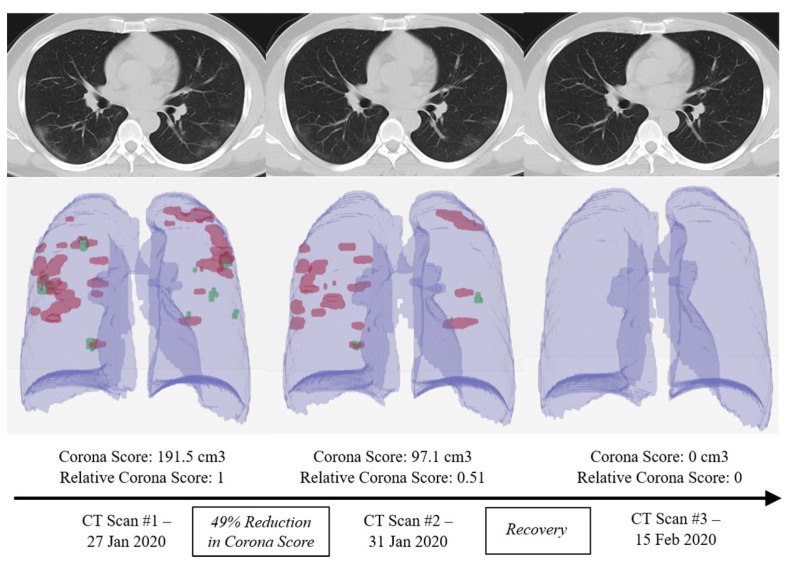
Using different computed tomography (CT) scans from a a COVID-19 patient, the RADLogics algorithm measures the recovery amount using a specific score [16].
